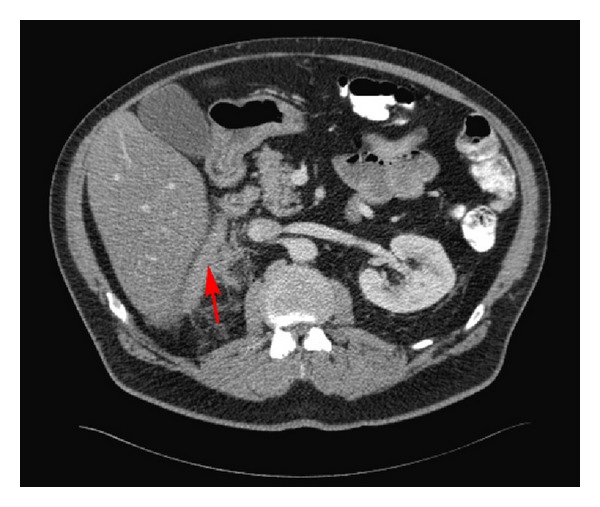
Abdominal computed tomography (CT) scan revealing streaky densities in the fat (red arrow) adjacent to the right adrenal gland; findings are consistent with hemorrhage.
